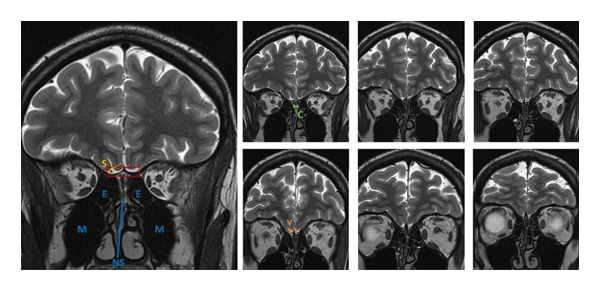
MRI scan with hypoplastic olfactory bulbs, normal depth olfactory clefts and minor inflammatory changes in the ethmoid sinuses. Note: S: olfactory sulcus; E: ethmoid sinuses; M: maxillary sinuses; NS: nasal septum; C: olfactory clefts; V: vessels; red rectangle: typical location of the olfactory bulbs, although they are not visible in this case.
